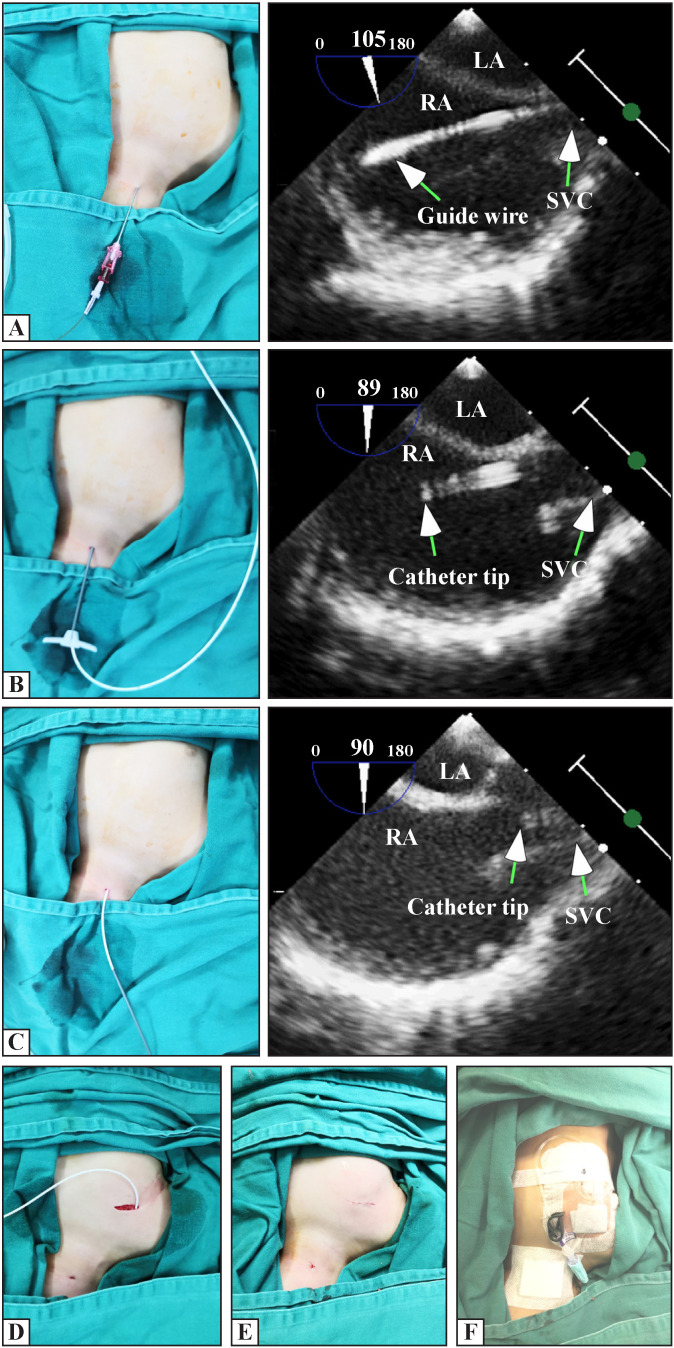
Transesophageal echocardiography-guided port catheter implantation. (A) The guide wire was in the RA. (B) The port catheter was inserted into the RA. (C) The catheter tip was placed at the level of the cavoatrial junction. (D) A port catheter was drawn, crossing above the clavicle using a tunnel needle. (E) The skin incision was closed by continuous intracutaneous suture. (F) Picture at the end of the operation. LA, left atrium, RA, right atrium; SVC, superior vena cava.
